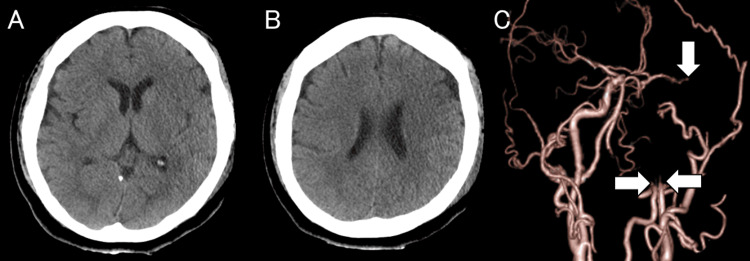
CT and three-dimensional CT angiography after neurological deterioration. Non-contrast head CT demonstrating subtle early ischemic changes in the left middle cerebral artery territory without intracranial hemorrhage (A, B). Three-dimensional CT angiography demonstrating occlusion of the left internal carotid artery, left vertebral artery, and left middle cerebral artery (arrows) (C).
